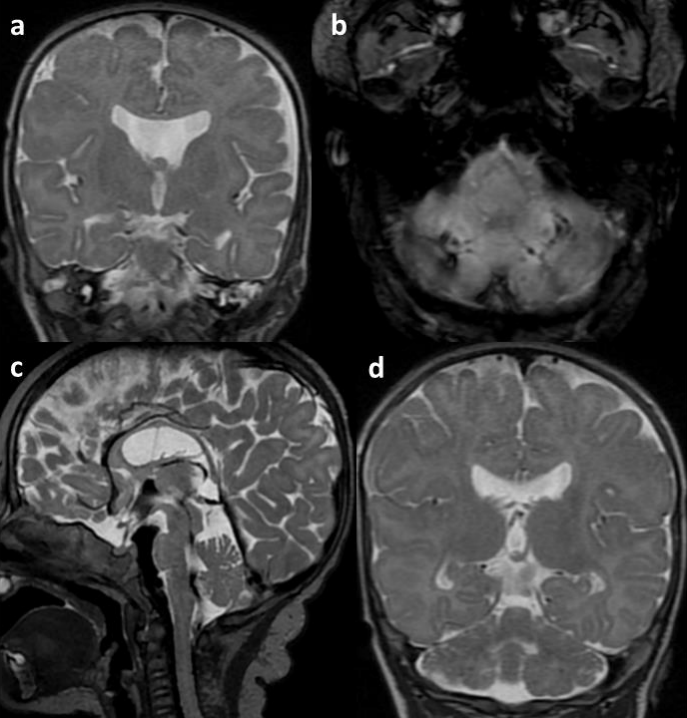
MRI of the brain at 14 weeks of corrected age. Lack of the septum pellucidum (a). Posthaemorrhagic haemosiderin deposits – black dots – in both cerebellar hemispheres on SWI sequence (b). Abnormal proportions of the cerebrum and cerebellum with decreased cerebellar volume on sagittal (c) and coronal (d) T2-weighted images.
